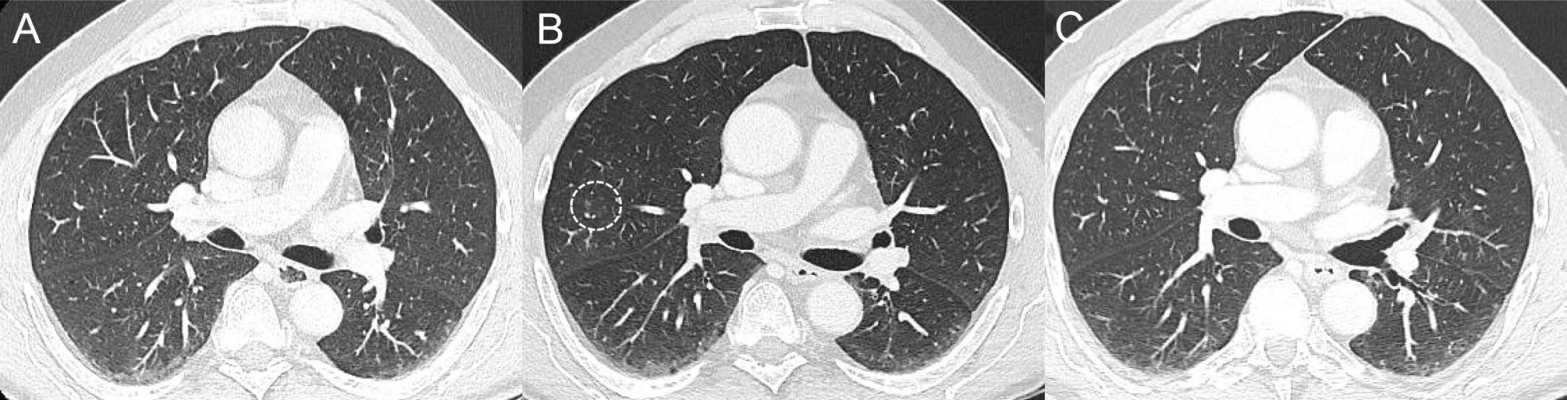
Three CT scans performed before and a few months after starting selpercatinib. (A) negative lung CT of the patient in April 2021, before starting selpercatinib. (B) evidence of a few centrilobular micronodules in the upper right lung lobe (white circle) in a CT scan of June 2021. (C) CT scan of August 2021 showing the complete resolution of the micronodules.
